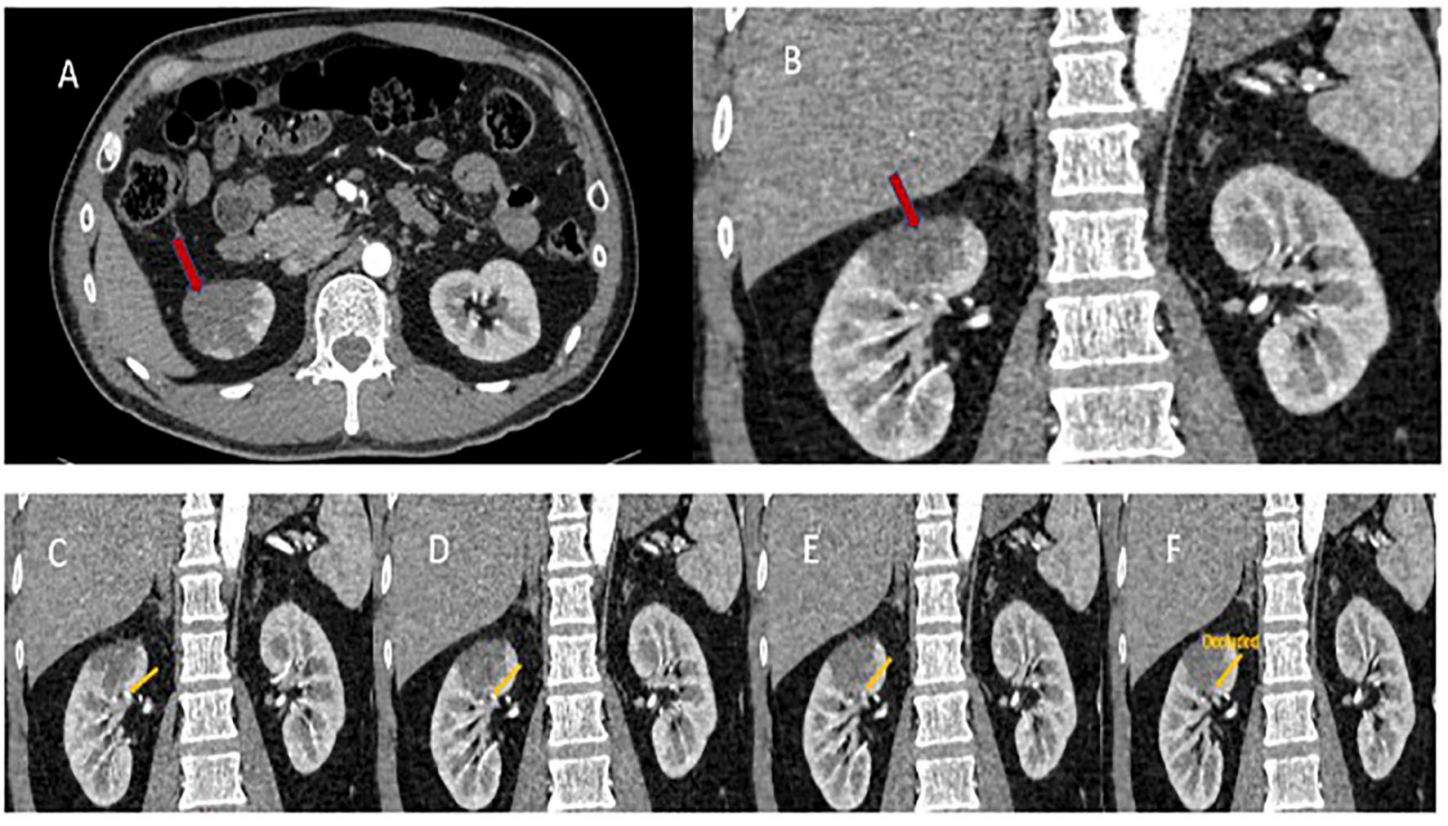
CT angiogram of the pelvis. CT angiogram of the pelvis showing areas of decreased attenuation (red arrows) on the axial (A) and sagittal (B) views, signifying infarction of the right kidney upper pole. Sequential flow of contrast (C–F) shows the absence of contrast blood in the proximal renal artery branch supplying the upper pole of the right kidney (yellow arrow), indicating occlusion of the artery.
